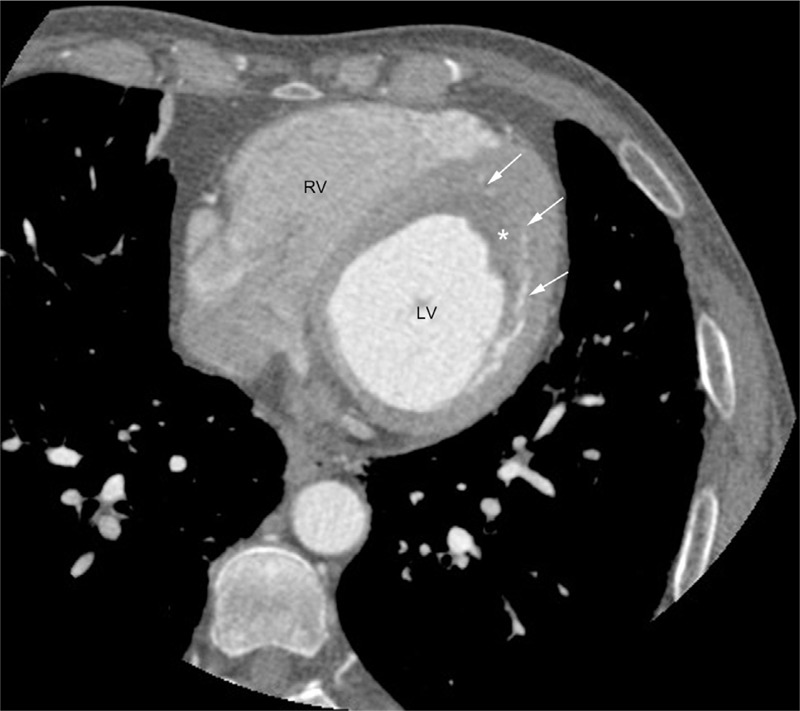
Images of conventional 64-row MSCT of the first patient. Image of the diastolic short axis shows a low-density shadow of filling defects at the LV apex (∗ delineates the contour of the thrombus; white arrows indicate the borders of the thrombus). LV = left ventricular, MSCT = multislice spiral computed tomography.
